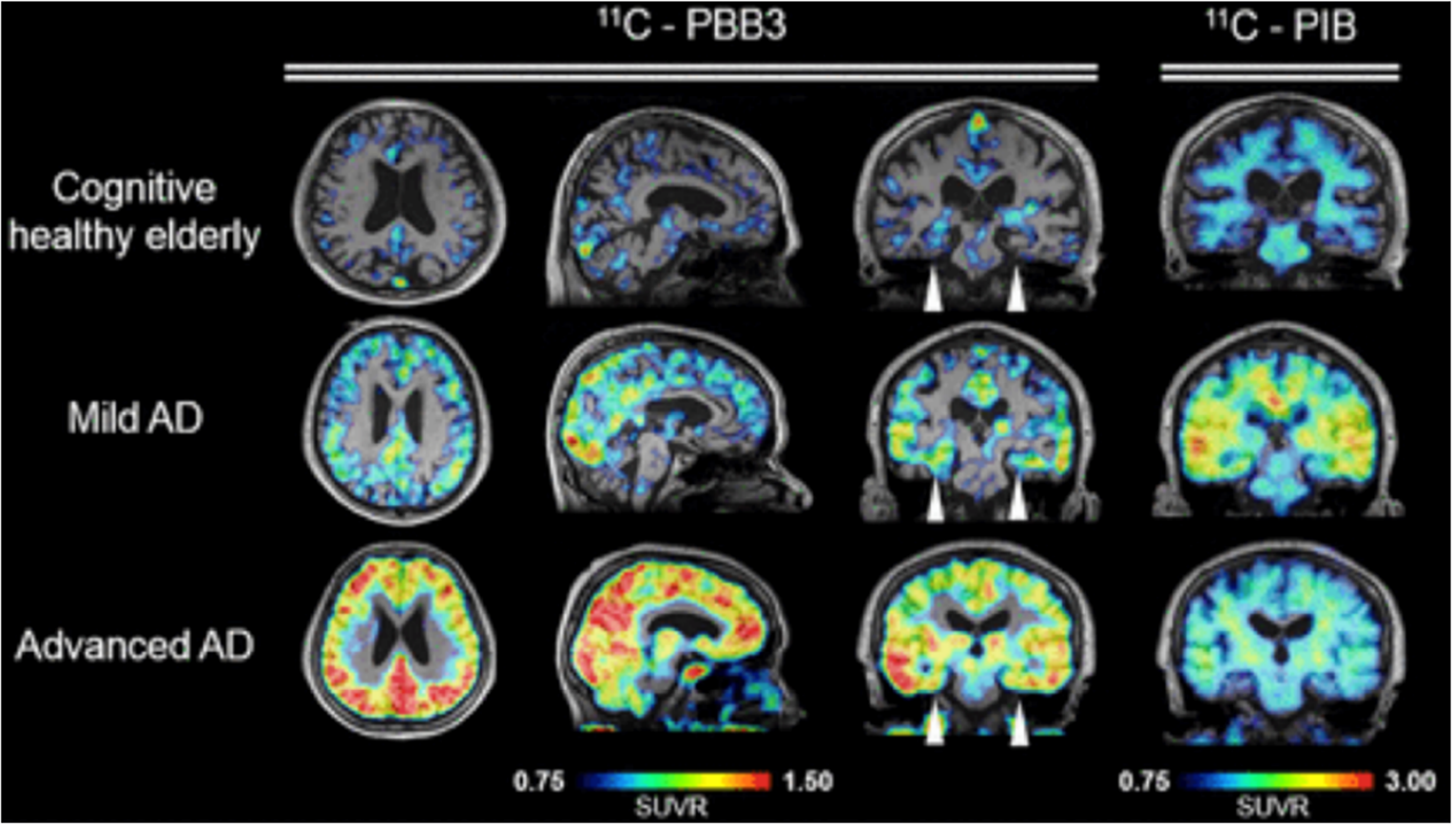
[^11^C]PBB3 PET images. [^11^C]PBB3 parametric standardised uptake value ratio (SUVR) images compared with [^11^C]PiB. [^11^C]PBB3 shows tracer uptake in cortical areas in advanced AD patient. This research was originally published in *JNM*. Shah M, Catafau AM. Molecular Imaging Insights into Neurodegeneration: Focus on Tau PET Radiotracers. J. Nucl. Med. 2014;55:871–874. © SNMMI [21]
